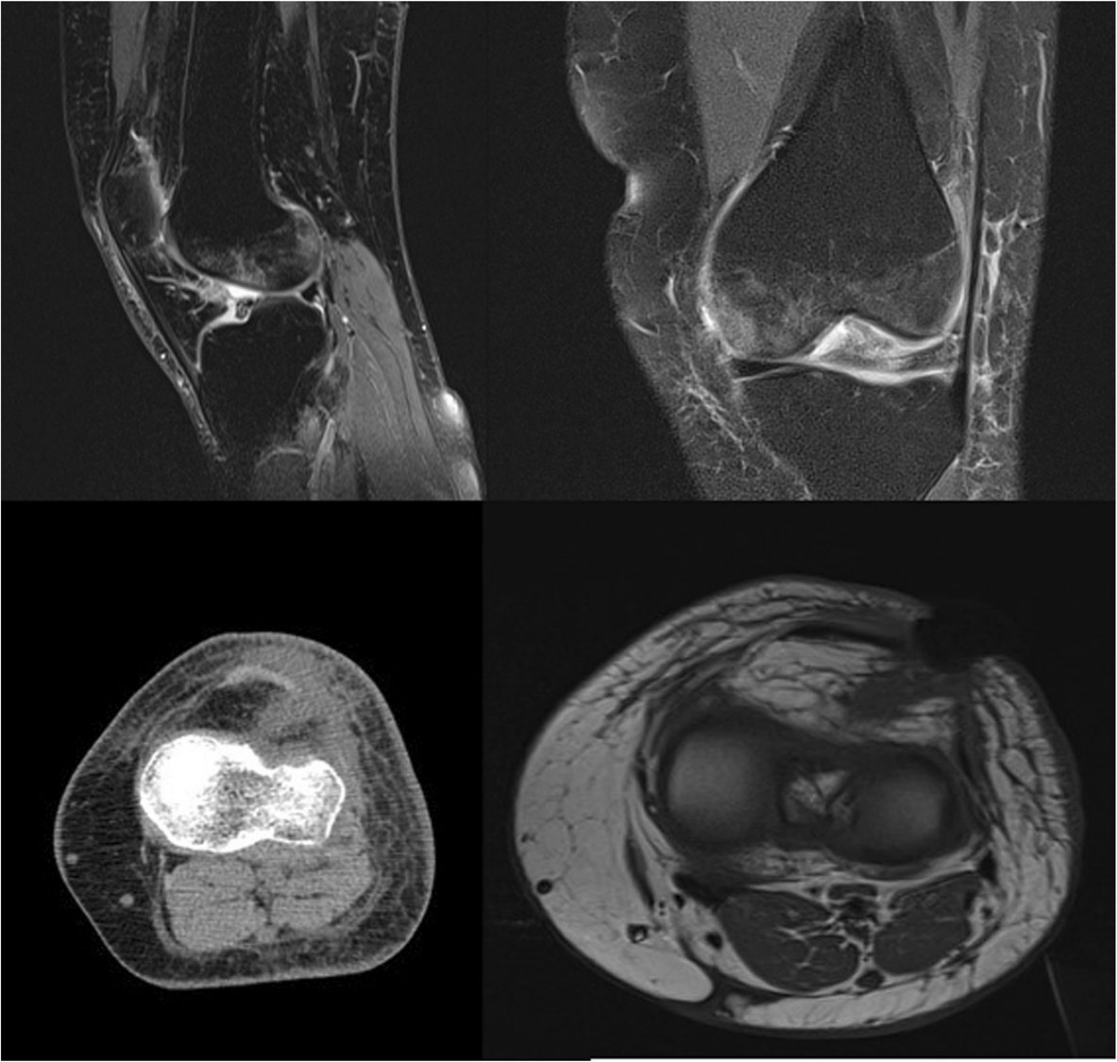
The MRI study of the knee shows signs of septic arthritis, with bone edema and abscesses around surgical site and next to Hoffa. The presence of imbibition and swelling of the periarticular tissues (cutaneous and subcutaneous) can be observed
